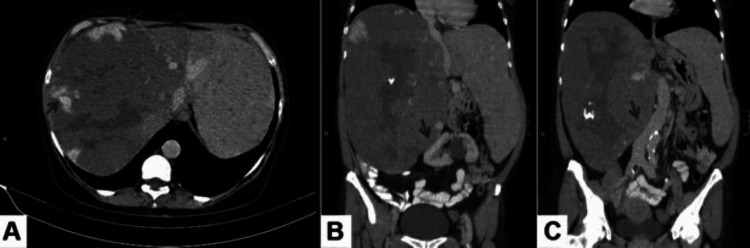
CECT scan of the abdomen in axial (A) and coronal (B, C) sections. The lesion measures 25x15 cm, which shows nodular enhancement in the periphery corresponding to the blood pool (grey arrow) CECT: contrast-enhanced computed tomography
